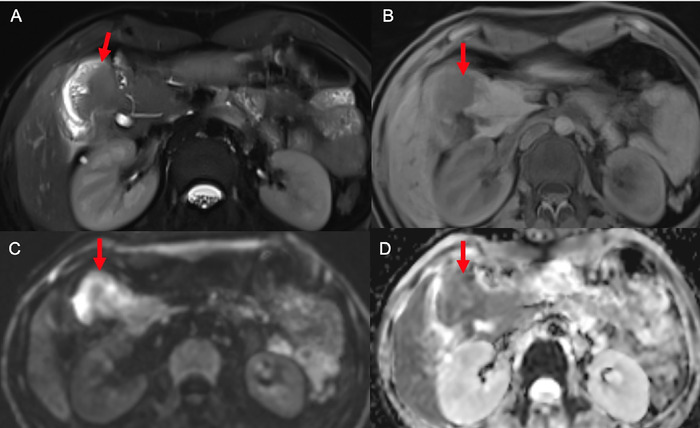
Magnetic resonance imaging of the upper abdomen. Duodenal mass (red arrows) appears isointense on (A) axial T2-weighted image and hypointense on (B) T1-weighted image. Diffusion restriction is seen in (C) diffusion-weighted image and corresponding (D) apparent diffusion coefficient map.
